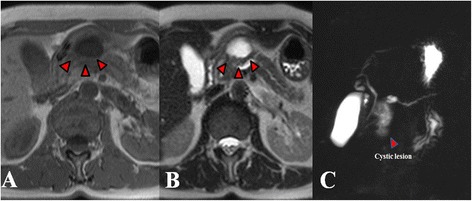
MRI. The massive simple cyst in the pancreatic body showed low signal intensity on A) T1-weighted MRI and B) high intensity on T2-weighted MRI. C) MRCP showed slight dilatation of the main pancreatic duct but stenosis of the duct of Wirsung was not seen.
